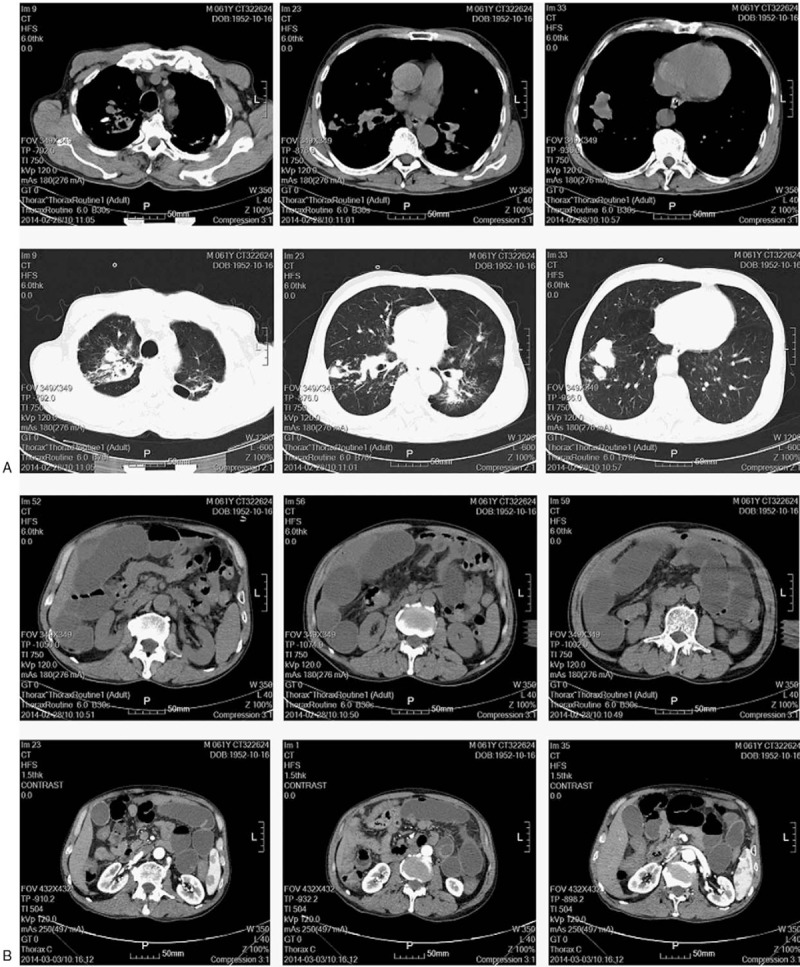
CT scans of chest and abdomen on hospital admission. (A) CT scans of the chest showed bilateral upper-lobe with patchy infiltrates, focal calcification, and nodular opacities peripherally with cavitation. (B) CT scans of the abdomen showed air-fluid levels in loops of small bowel.
